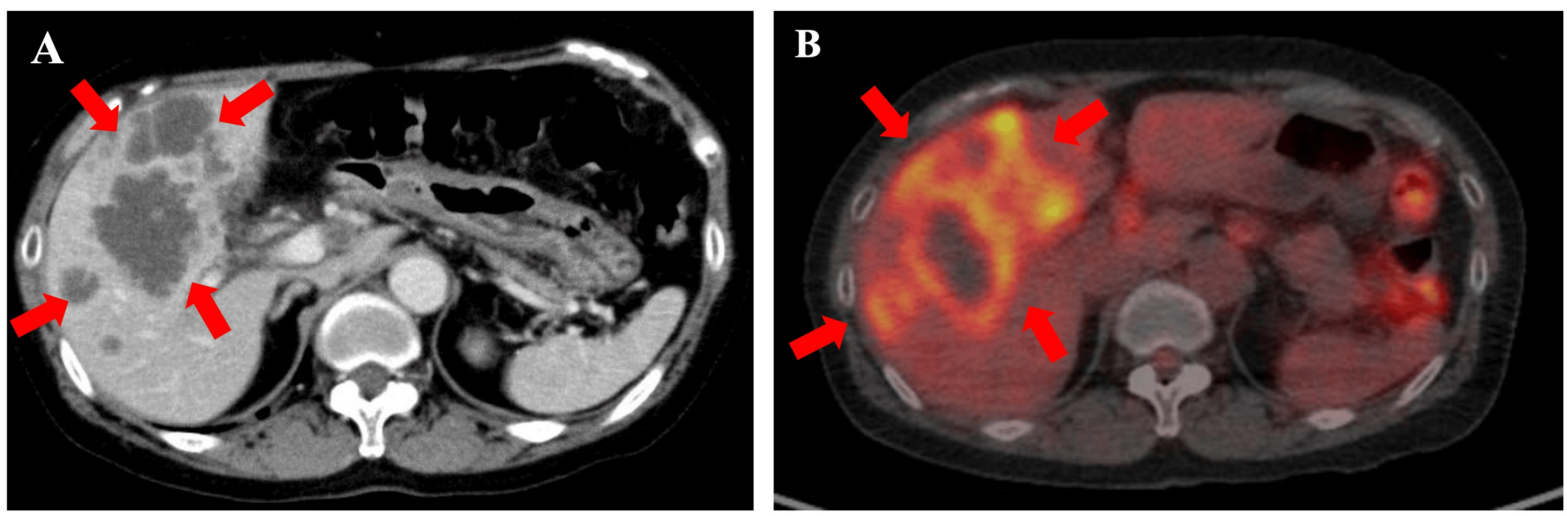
Baseline CT and PET-CT images of the abdomen before treatment (A) CT scan showing a large, predominantly hypodense mass measuring 10 × 8.4 cm in the right hepatic lobe at presentation. (B) Baseline PET-CT scan demonstrating a nodule in the right hepatic lobe with increased uptake of ¹⁸F-FDG. FDG, fluorodeoxyglucose
